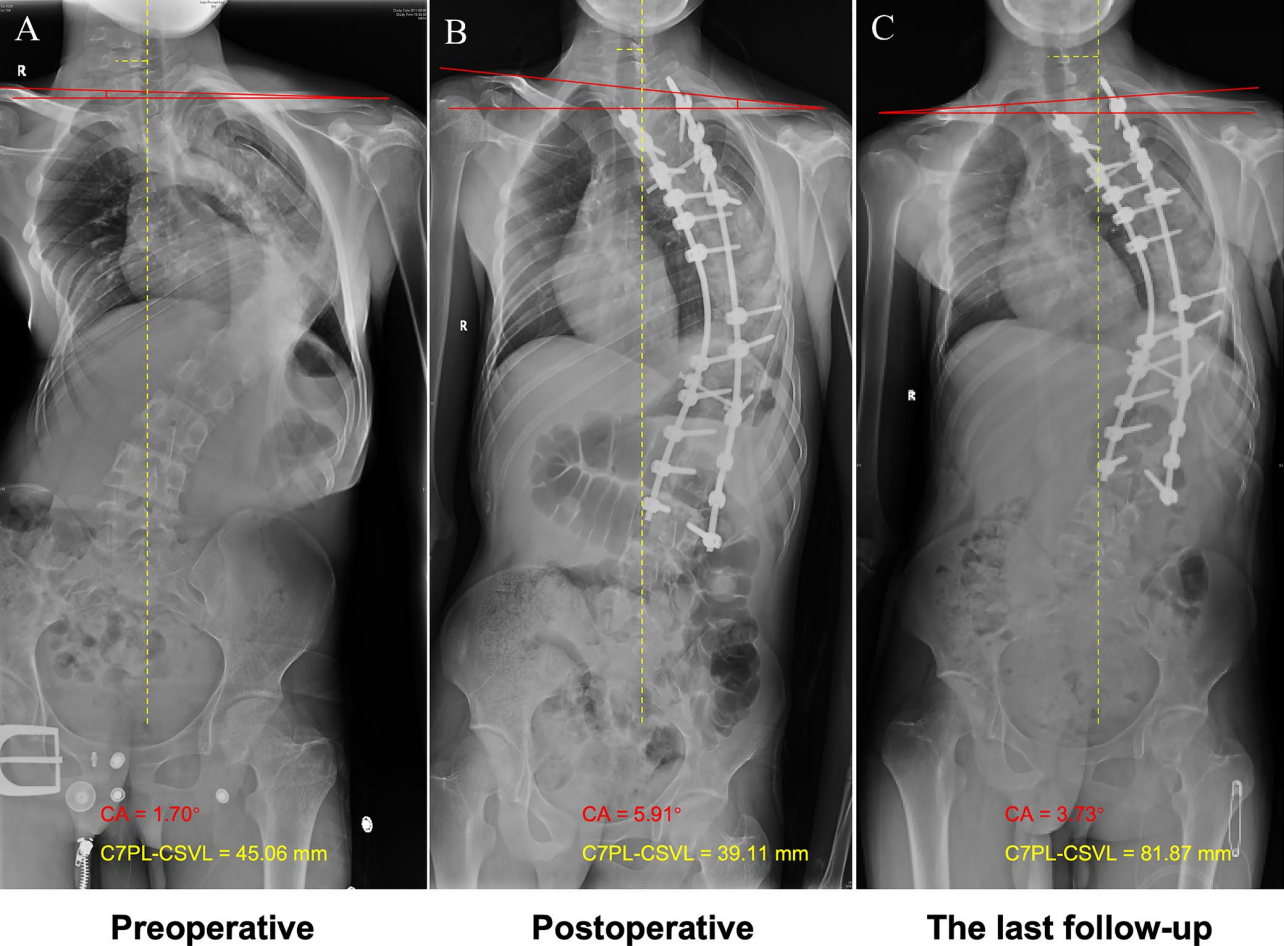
The preoperative, immediately postoperative, and the last follow‐up radiographs of a representative case. Posteroanterior radiographs were obtained in a 13‐year‐old female who was diagnosed with severe and rigid congenital scoliosis and underwent posterior instrumentation. Shoulder imbalance and coronal imbalance were observed preoperatively, and the C7PL‐CSVL was slightly corrected by surgery (45.06 mm to 39.11 mm). However, the patient developed shoulder imbalance (CA = 5.91°) towards the opposite side postoperatively. During the follow‐up, the aggravated coronal imbalance (C7PL‐CSVL = 81.87 mm) was observed, C7PL shifted towards the side of higher shoulder, and the shoulder imbalance was improved (CA = 3.73°).
